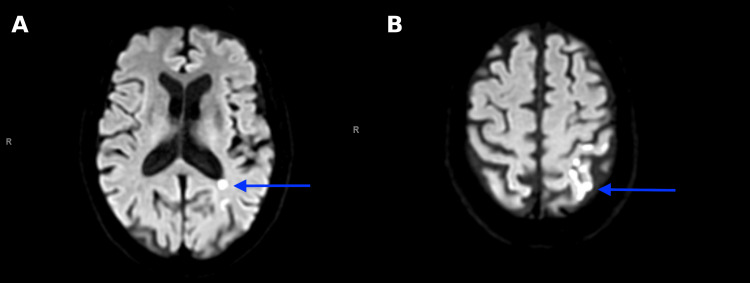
MRI brain axial diffusion weighted imaging sequence (A) Area of restricted diffusion at the periatrial region of the left lateral ventricle; (B) Areas of restricted diffusion at the left parietal lobe
